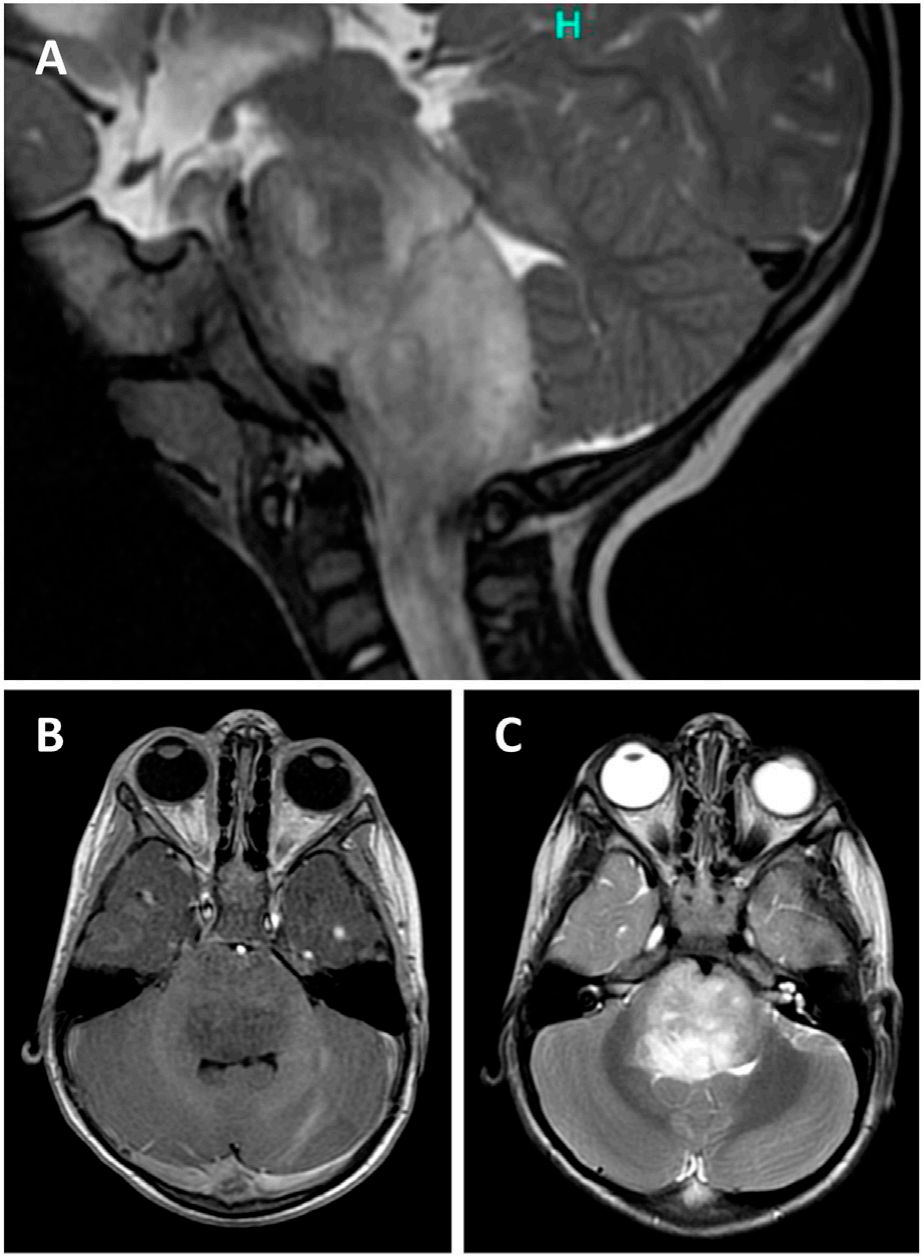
Sagittal T2-weighted (T2W) magnetic resonance image (MRI) of the posterior fossa showing a partly exophytic mass lesion in the pontine and medullary regions with 30 mm axial and 49 mm cranio-caudal greatest extension (A). The tumour is hypointense on T1W (A) and hyperintense on T2W (B) imaging without significant contrast enhancement.
